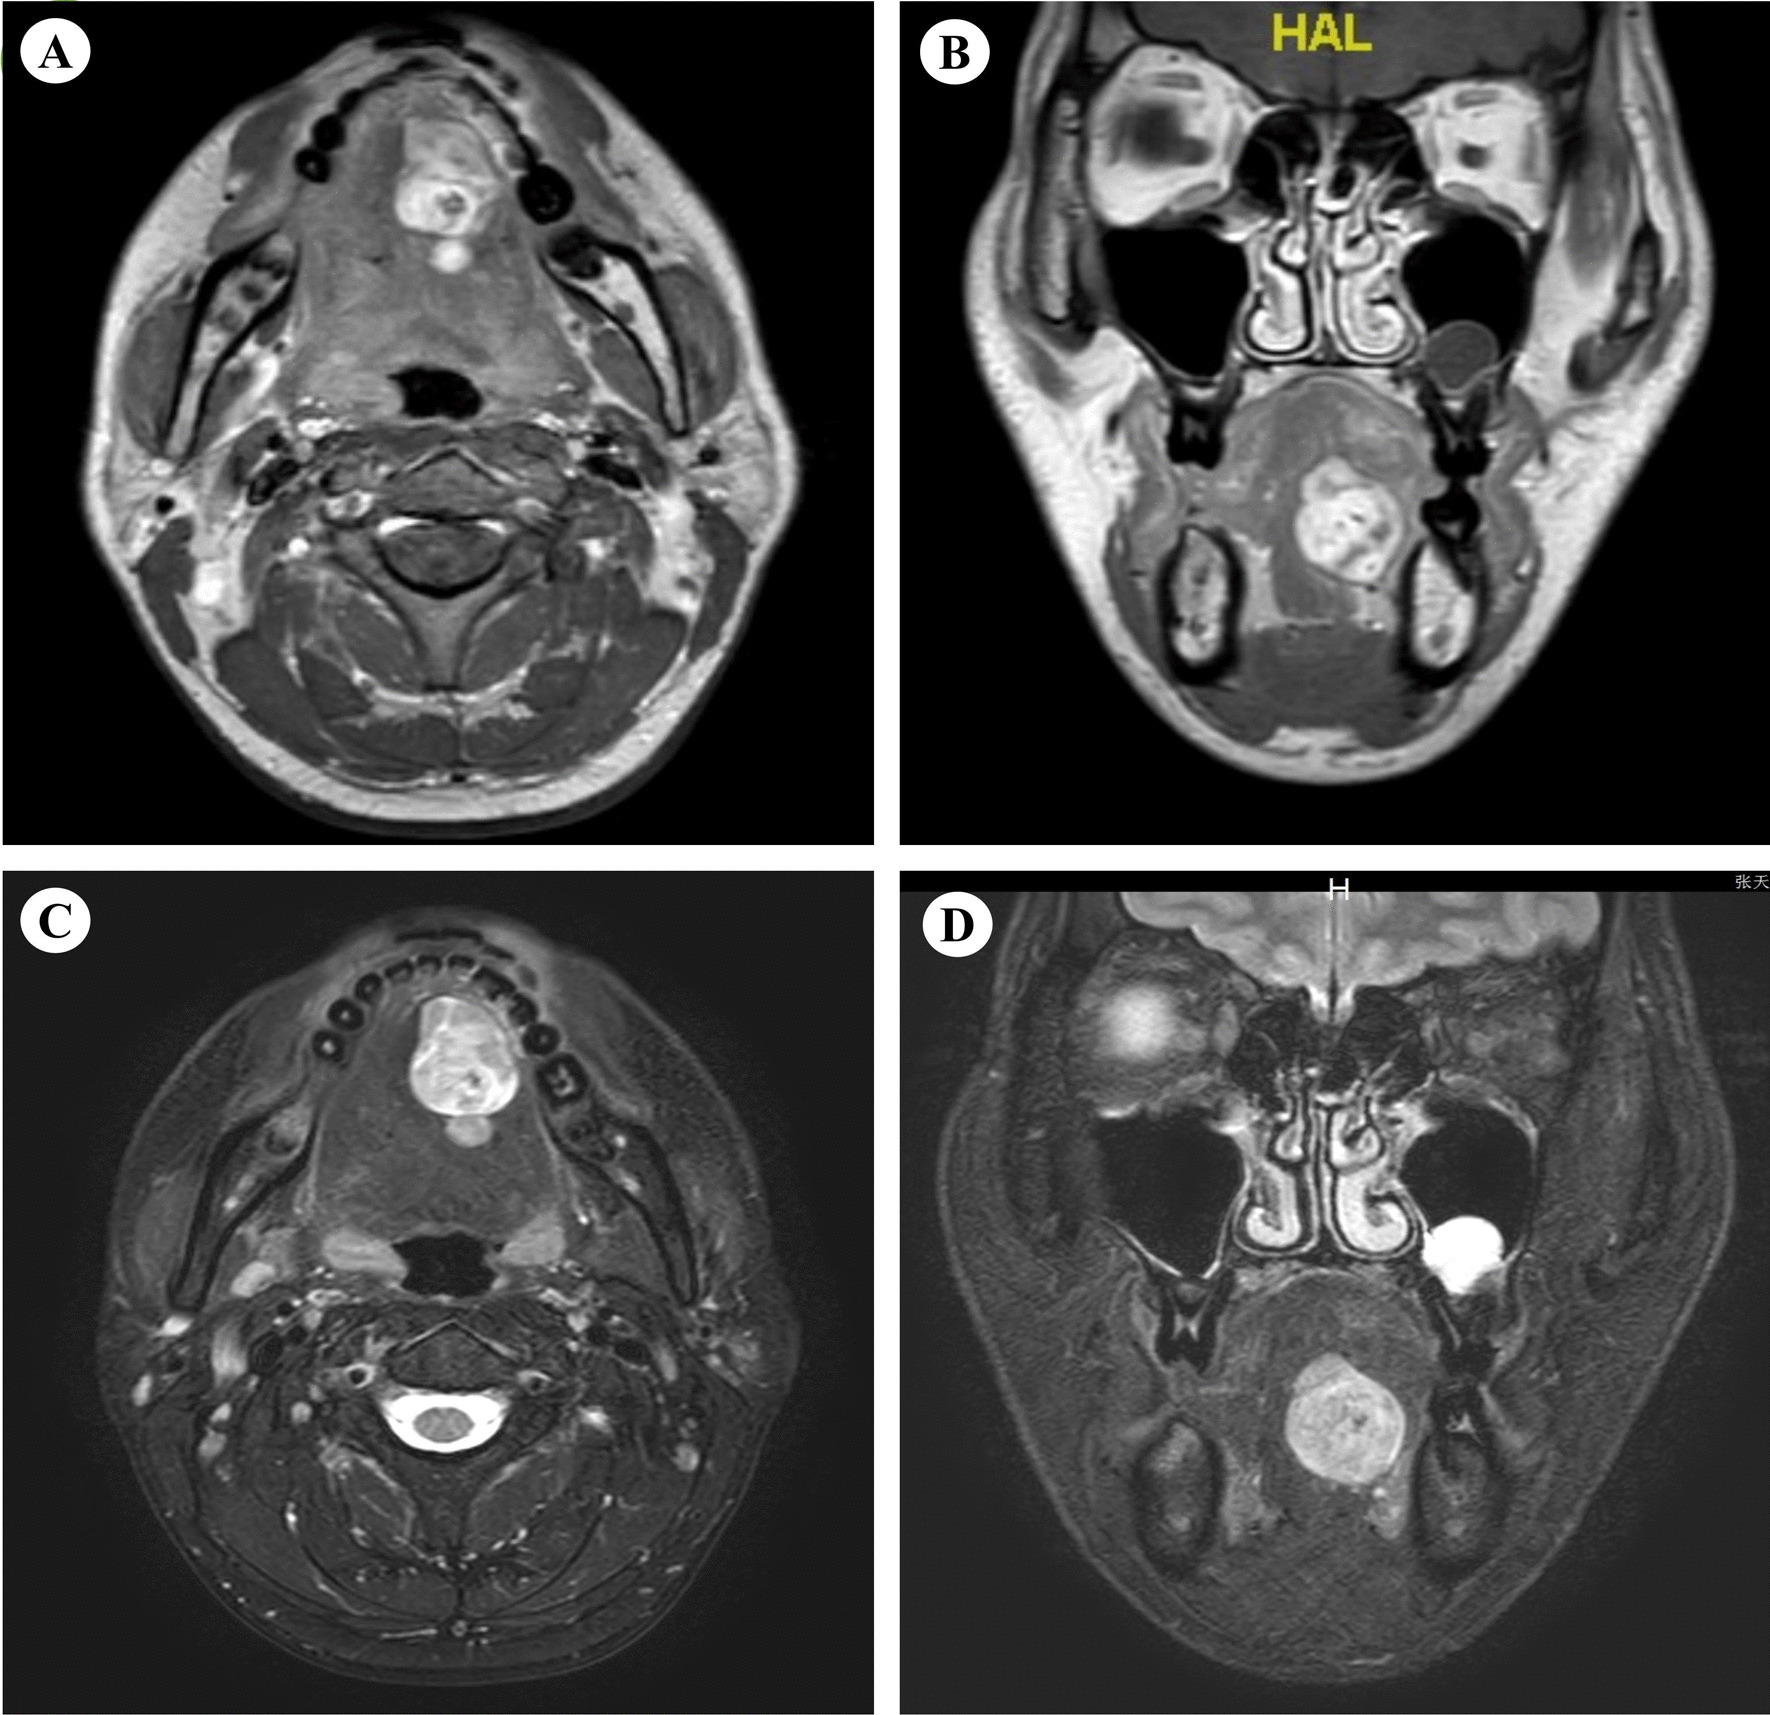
The tumor displayed a heterogeneously equal signal on T1-weighted images (A, B), and showed a heterogeneously high signal on T2-weighted images (C, D)
